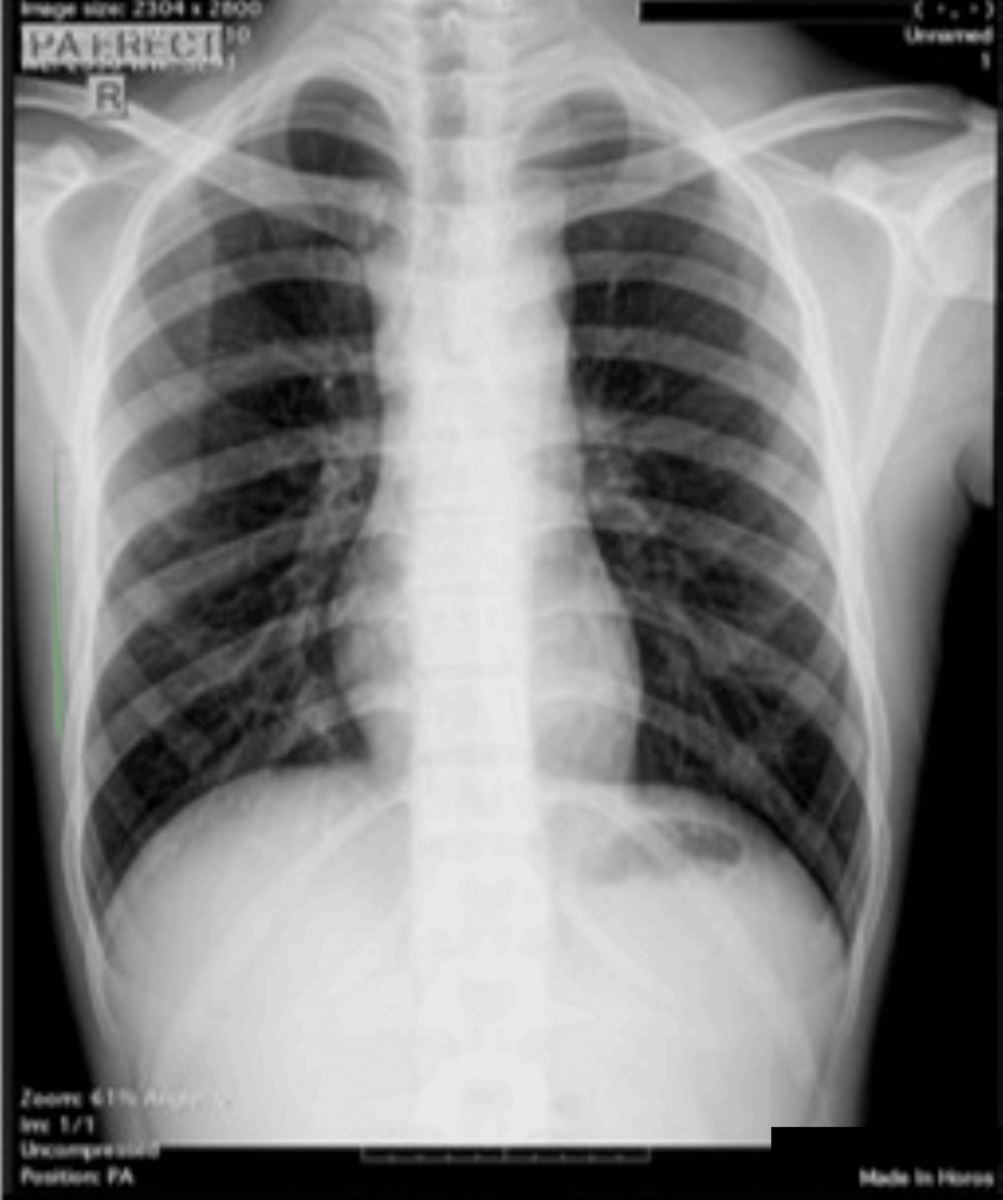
Chest x-ray before treatment showed no abnormality
